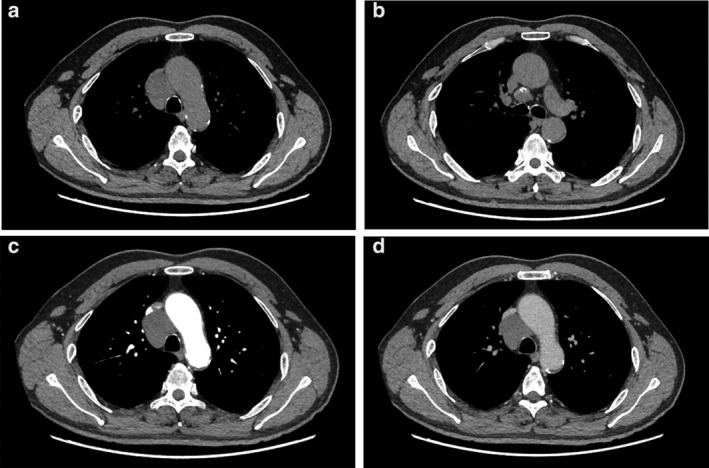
(a) An irregular slightly low‐density shadow appeared in the anterior trachea and posterior superior vena cava of the mediastinum on chest enhanced computed tomography (CT). The lesion boundary was clear. (b) A small calcification and solid component were visualized at the lower edge of the lesion. The lesion measured 5.3 cm × 4.4 cm × 4.8 cm (upper and lower × front and rear × left and right), and the scan CT value was approximately 25 HU, enhanced CT value approximately 36 HU. (c,d) A small piece of solid component was clearly enhanced.
